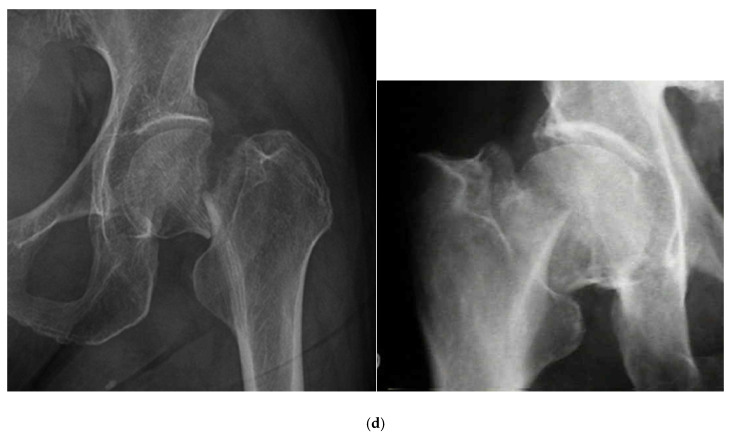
Example radiographs demonstrating Garden classification: (a) Garden I; (b) Garden II; (c) Garden III; (d) Garden IV.
